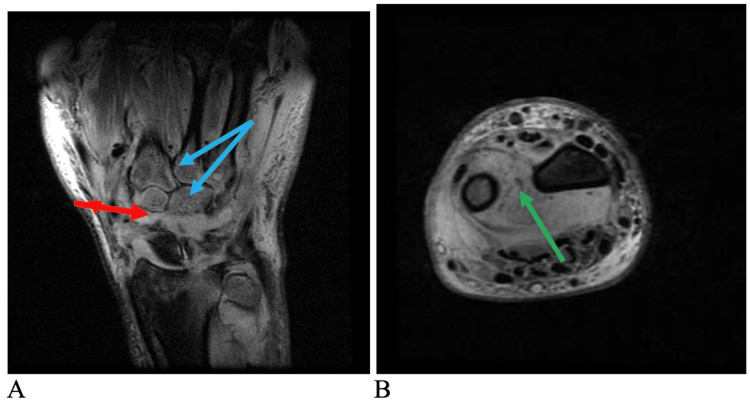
Preoperative MRI of the left wrist The images demonstrate abnormal signal intensity in the intercarpal region (red arrow). Bone marrow edema is identified in the proximal and distal carpal row as well as the 3rd to 5th metacarpal bases (blue arrows) (A). Synovitis is seen in the distal radio-ulnar joint and in the pronator quadratus (green arrow) (B) MRI: magnetic resonance imaging
